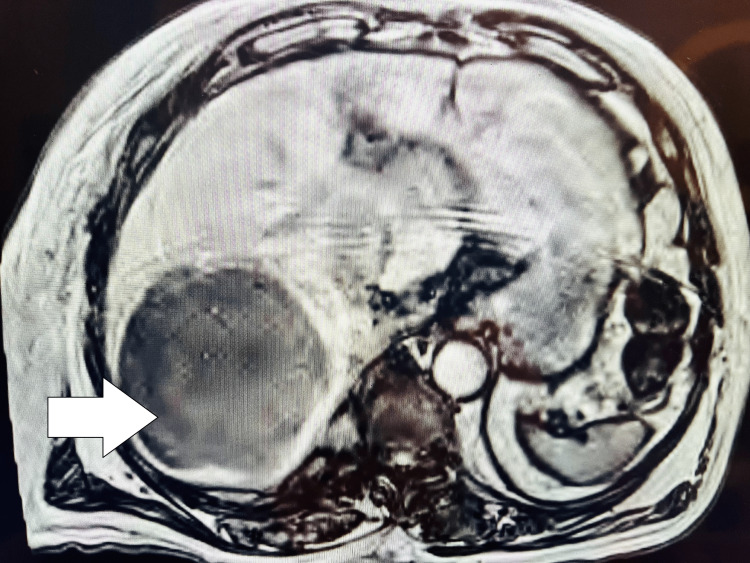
MRI image of necrotic liver metastasis
